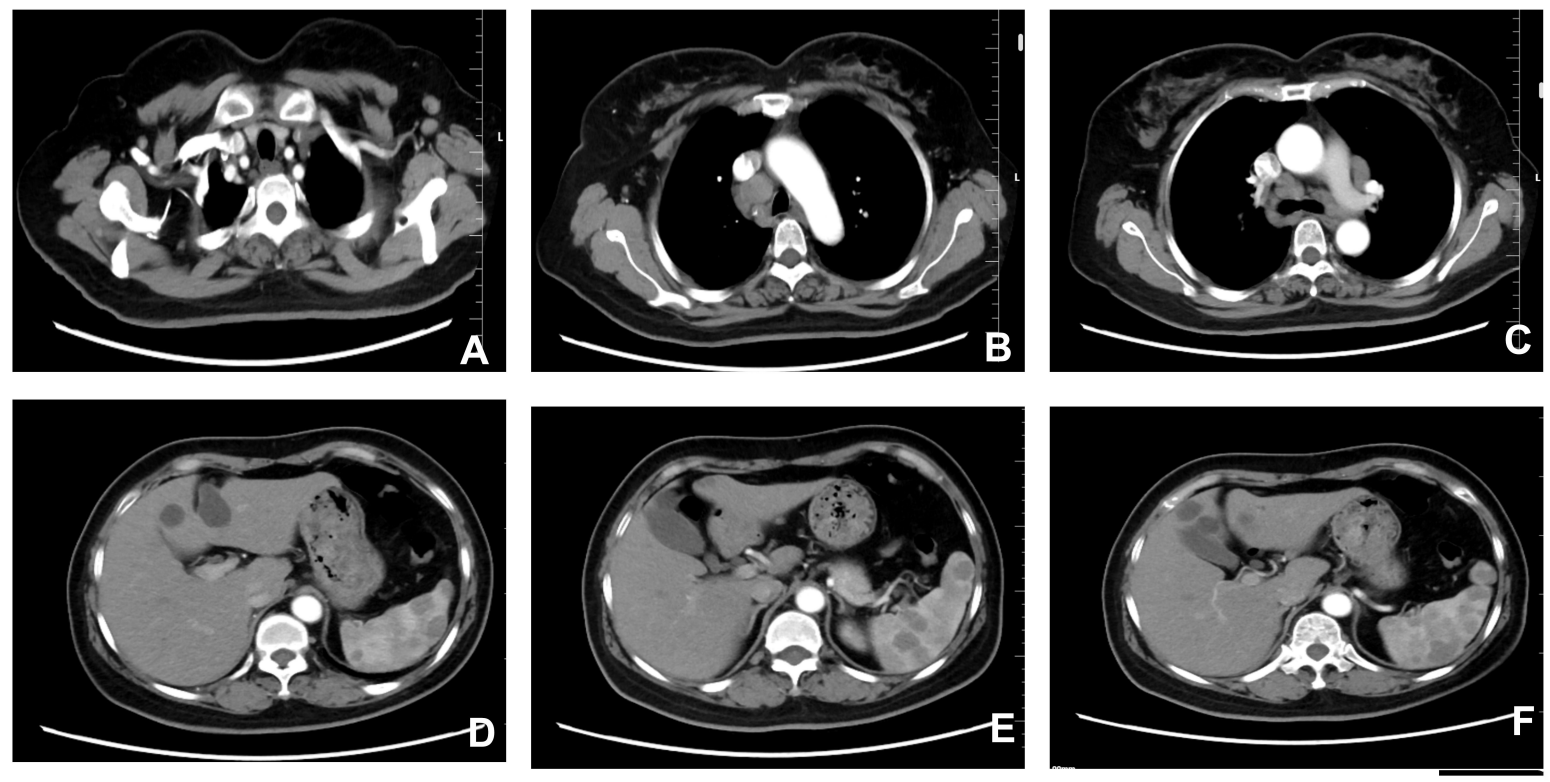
Chest and upper abdominal CT. (A) Thoracic inlet level. (B) Aortic arch level. (C) Carina level. (D) Gastric body level. (E) Splenic artery level. (F) Splenic level. Scale bar = 2 cm.
